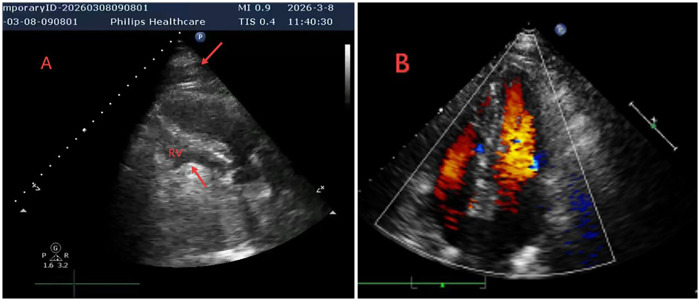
(A) Echocardiography shows a large right ventricular hematoma (arrow) with mild pericardial effusion (arrow). (B) Echocardiography shows resolution of the hematoma, with the right atrium and right ventricle returning to normal size.
